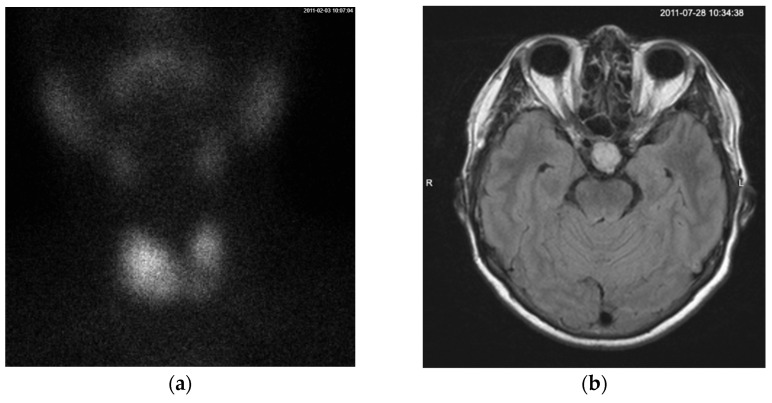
(a) On the Tc99 SPECT scan, the thyroid gland is in its typical anatomical position. The functional distribution is asymmetrical, with the left lobe smaller than the right lobe. Radiotracer uptake is observed on both sides, with more intense uptake in the lower two-thirds of the right lobe extending toward the isthmus. A pyramidal lobe uptake is visible superior to the isthmus. (b) MRI contrast enhanced T1-weighted axial scan showing a mass measuring 17 × 20 × 18 mm in the sella turcica region that is moderately enhanced by contrast material without a mass-compression effect on the surrounding structures, consistent with a macroadenoma. A cystic mass with well-defined borders in the right maxillary sinus was also noted.
